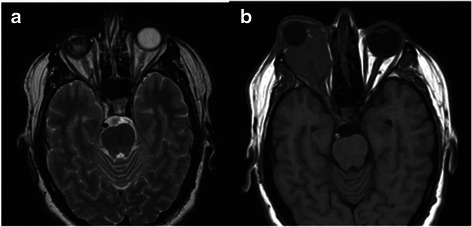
Magnetic resonance imaging scans showing a the atrophic right eye and b a large mass occupying the entire right socket displacing the prosthesis, reaching the optic foramen and eroding the bone wall of orbit and ethmoid
